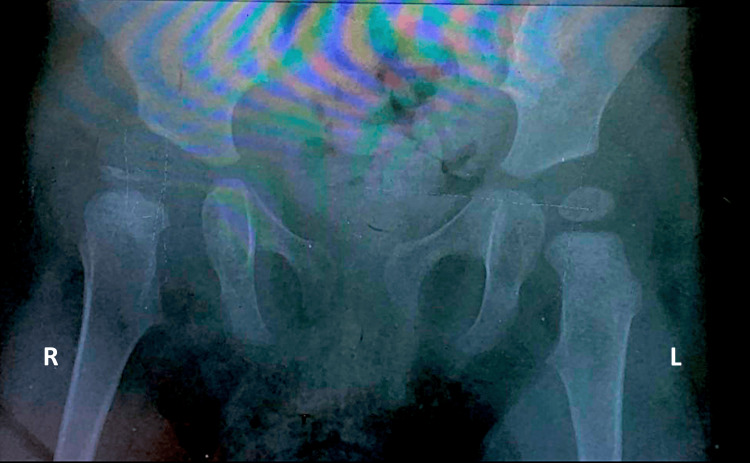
Follow-up radiograph demonstrating persistent acetabular dysplasia, hip subluxation, and fragmentation of the femoral head ossific nucleus (Tönnis and Kuhlmann type III). R: right. L: left
